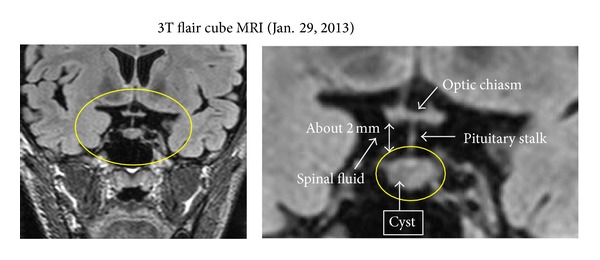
The 3T flair cube MRI taken on January 29, 2013. The MRI images demonstrated a RCC in the right pituitary region.
